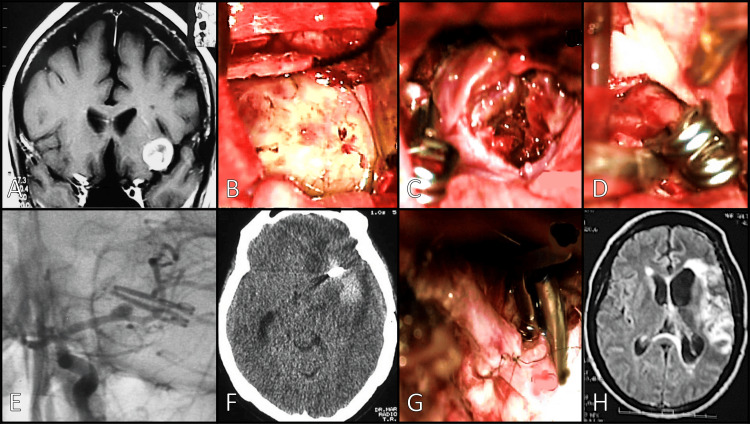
Case 3. (A) MRI coronal view showing a giant saccular aneurysm in left MCA. (B) Intraoperative exposure of the dome. (C) Aneurysmectomy and evacuation of the thrombus. (D) Two straight clips and a residual aneurysm were observed. (E) Postoperative DSA showing two straight clips and residual aneurysm. (F) Brain CT showed a new SAH episode with a surrounding hematoma in the left MCA. (G) MI-M2 end-to-end anastomosis. (H) Postoperative brain MRI MRI: magnetic resonance imaging; SAH: subarachnoid hemorrhage; DSA: digital subtraction angiography; CT: computed tomography; MCA: middle cerebral artery
